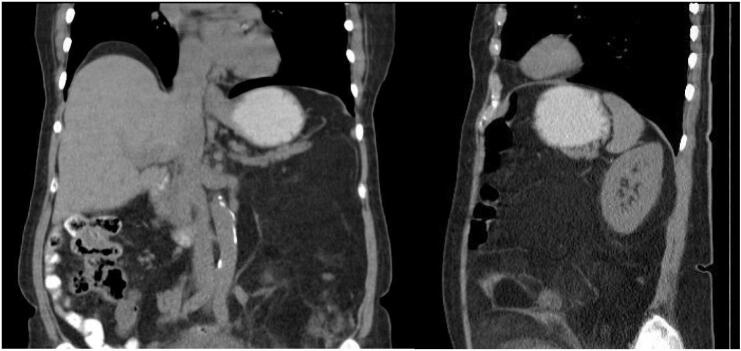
CT in coronal and sagittal views: a left para-aortic retroperitoneal mass is identified, compressing the descending colon against the anterior wall. In both views, the mass extends from the subphrenic region to the pelvis.
